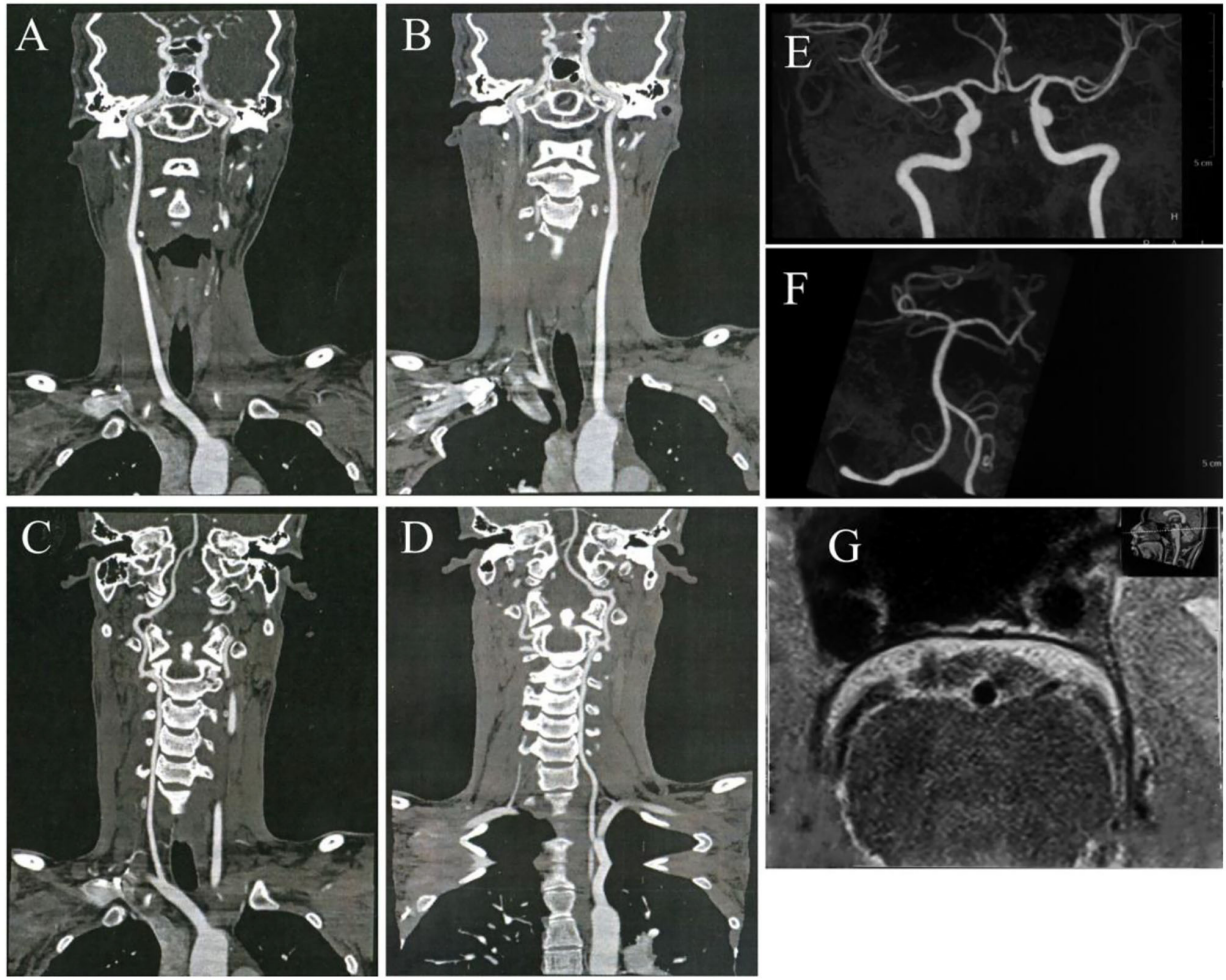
No arterial occlusion, stenosis, or plaque was discovered in bilateral carotid arteries (A,B), vertebral arteries (C,D), and all the intracranial arteries (E,F). The high-resolution vessel wall magnetic resonance imaging (MRI) of the basilar artery shows no occlusion or stenosis (G).
